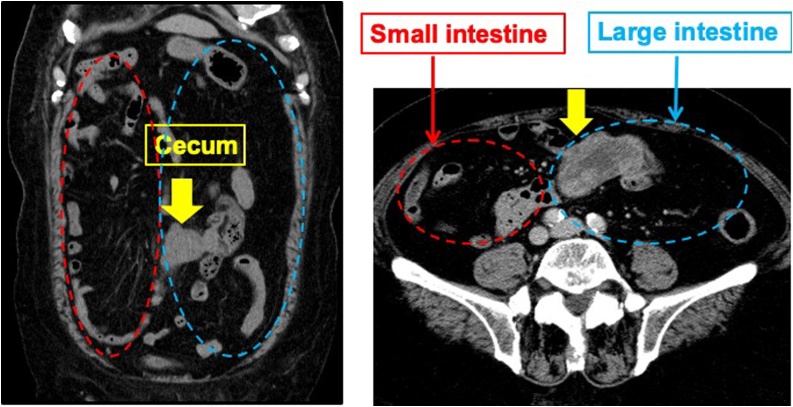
Contrast-enhanced CT. The images show the thickness of the appendiceal wall, which is located along the midline of the abdomen. The small intestine and colon are at the right and left sides of the abdominal cavity, respectively.
